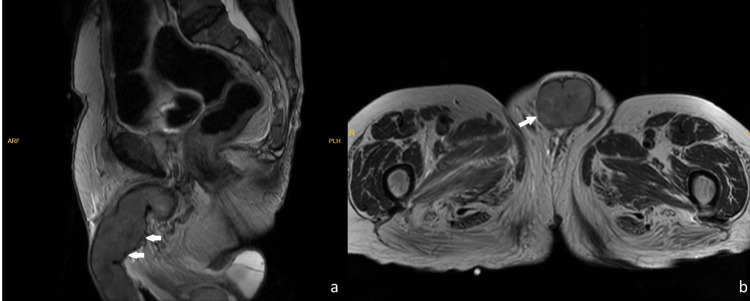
Pelvic magnetic resonance imaging of the patient. Penile squamous cell carcinoma invading the corpus cavernosum (the white arrows indicate the mass) on magnetic resonance imaging (MRI) (1.5-Tesla MRI (Signa, GE Medical Systems, USA)). a: Sagittal plane, b: Transverse plane.
